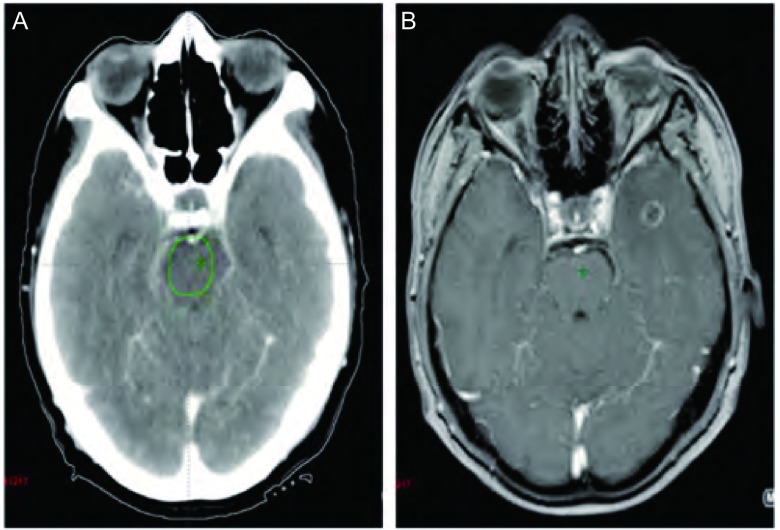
增强CT图像（A）和MRI图像（B） Enhanced CT image (A) and MRI image (B)
